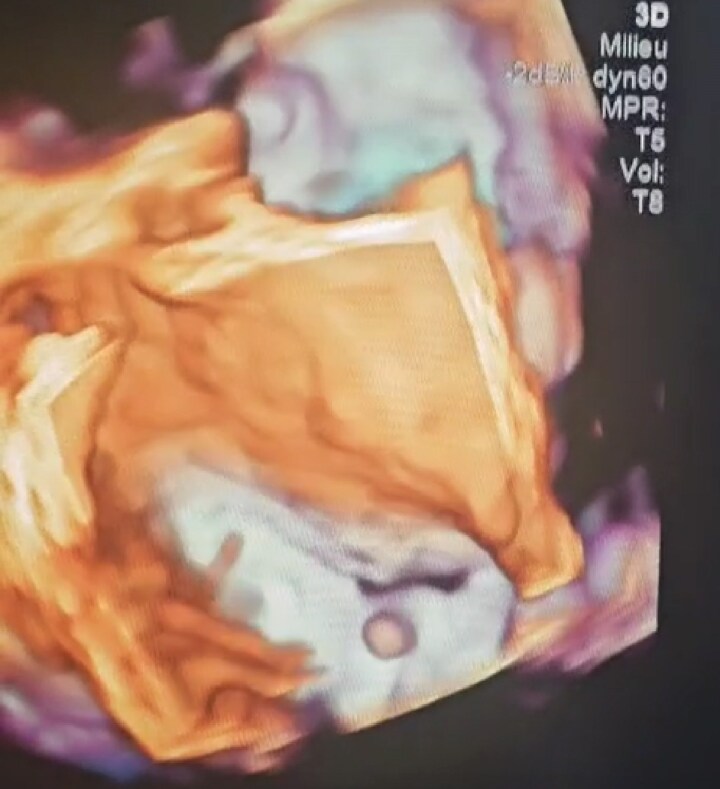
Three-dimensional transoesophageal echocardiographic view: a filamentous mass attached to the atrial surface of the mitral valve. The mitral leaflets are structurally preserved.
